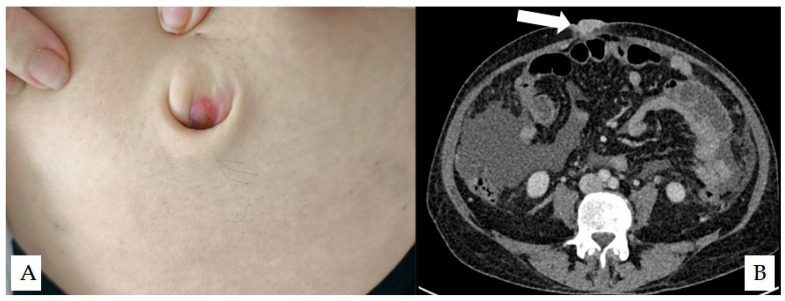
Clinical and radiological findings. (A) Umbilicus everted by the patient’s fingers demonstrating a small nodular lesion within the umbilical fold. (B) Axial contrast-enhanced computed tomography image showing metastatic pancreatic cancer with ascites. On re-review, a subtle soft tissue nodule (arrow) replacing the umbilicus can be identified. Sister Mary Joseph nodules represent cutaneous metastases to the umbilicus and are most commonly associated with advanced intra-abdominal malignancies, particularly of gastrointestinal or gynecologic origin [1]. Classically, these lesions present as firm, irregular, and often prominent umbilical masses that may distort or replace the normal umbilical contour [2]. In contrast, the lesion in the present case was small, located within the umbilical fold, and did not result in gross deformation of the umbilicus. Although a variety of benign conditions (such as dermatofibromas or keloid scars) may present with similar appearances, interpretation within the appropriate clinical context is essential [3]. The differential diagnoses for umbilical lesions are broad and may include umbilical hernia, omphalitis, and endometriosis alongside metastatic lesions [4]. In patients presenting with red-flag features such as unexplained weight loss, jaundice, or abdominal pain, the presence of an umbilical lesion should prompt consideration of underlying intra-abdominal malignancy [5]. Even subtle findings may therefore warrant further evaluation. Such presentations may be easily overlooked without careful physical examination, including eversion of the umbilicus. The novelty of this case lies not in the diagnosis itself, but in the subtle “intrafold” presentation, which required eversion of the umbilicus and re-review of imaging for detection. Although management was unchanged in this case, the finding supports the importance of careful physical examination as part of the clinician’s diagnostic armamentarium.
